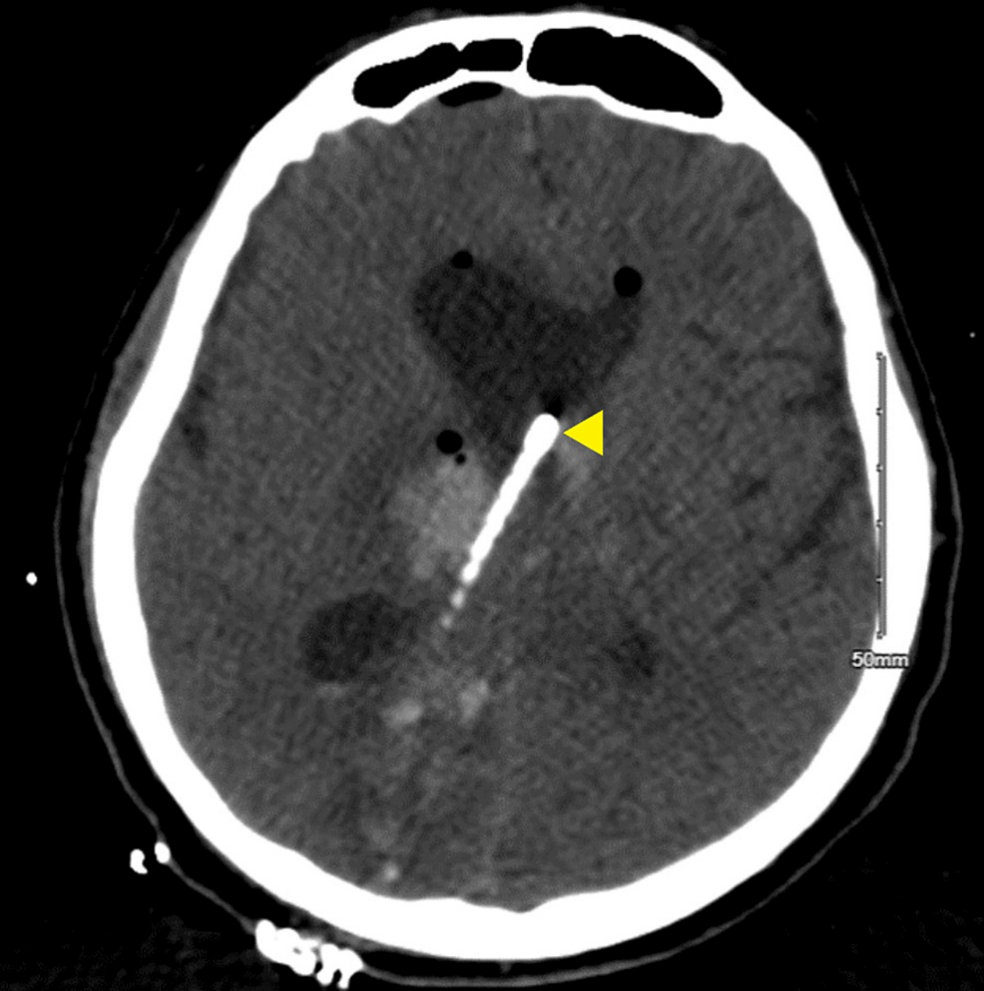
CT head without IV contrast on day 15 showing ventriculostomy placement in right ventricle CT head without IV contrast imaged on hospitalization day 15 showing ventriculostomy placement in the right ventricle for non-communicating hydrocephalus.
